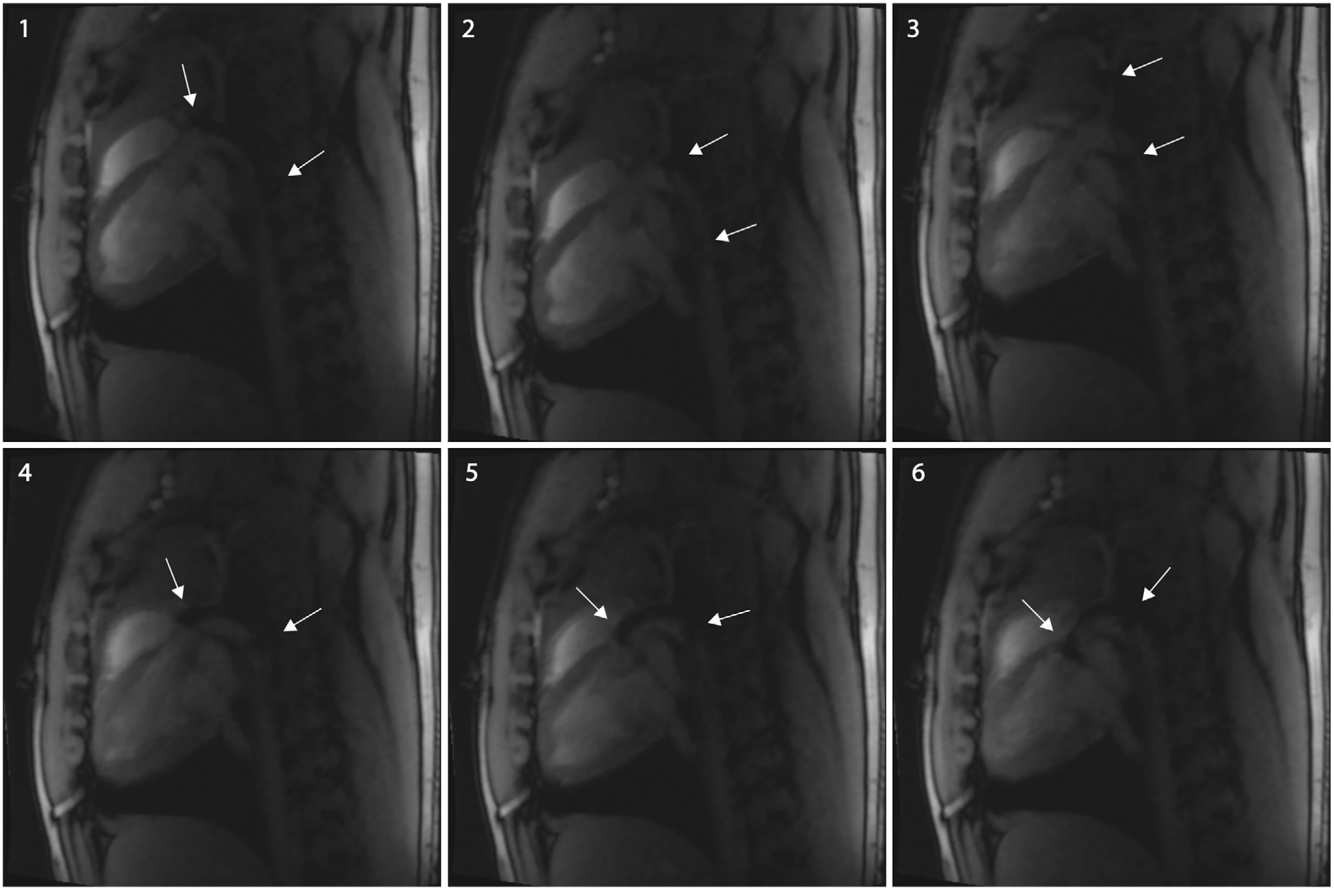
In vivo real‐time MRI of steerable catheter manipulation in a clinical 3 Tesla system. Arrows point at the two markers on the catheter tip. 1) Catheter advancing towards the aortic arch. 2,3) Catheter pointing towards the carotid artery. 4,5) Catheter steering towards the aortic arch. 6) Catheter crossing the aortic arch towards the aortic root.
